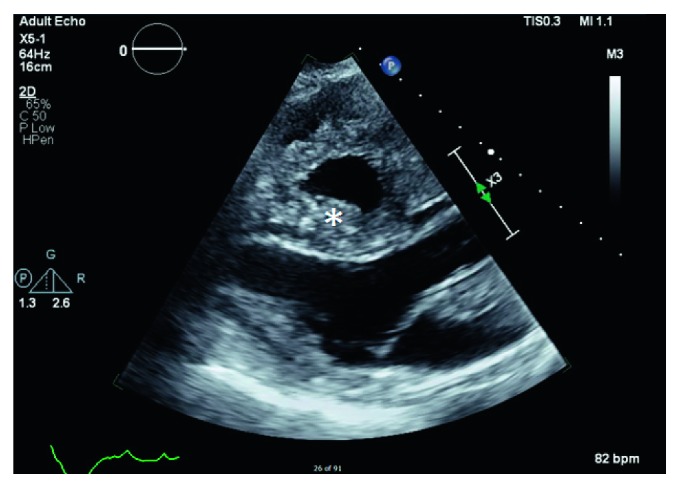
Transthoracic echo—parasternal long axis view. The cardiac cycle is in systole and the right ventricle fails to have concentric contraction demonstrated by the loss of curvature in the posterior right ventricle (^∗^). This indicates severe right ventricle dysfunction with severe hypokinesis/akinesis in the basal to apical wall due to infiltration. The cardiac tissue overall lacks any significant involvement of the posterior walls given the normal left atrium and left ventricle.
